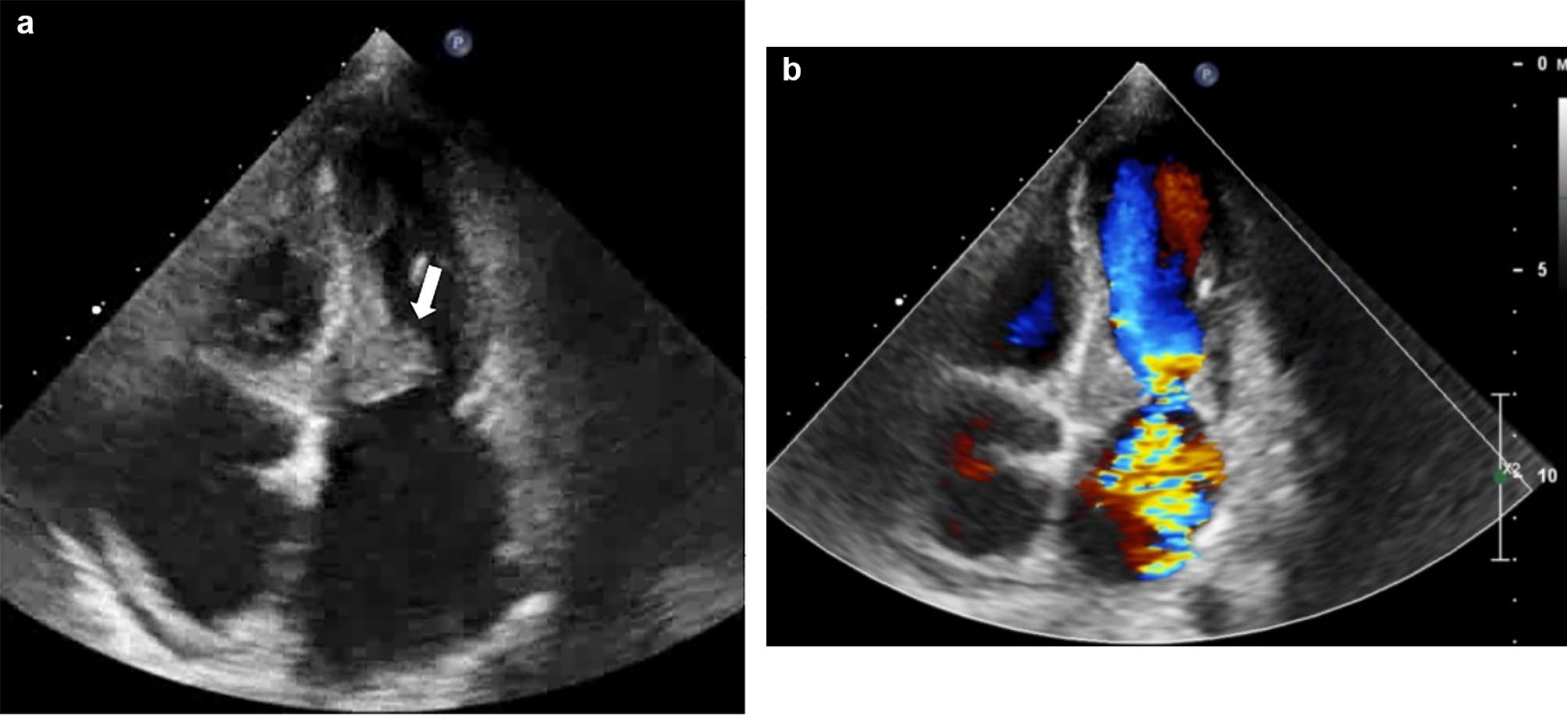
Apical 4-chamber view. An increased thickness in the ventricular side of mitral valve leaflets is seen (A, arrow), resulting in mass formation. In addition, severe mitral regurgitation is also present (B)
